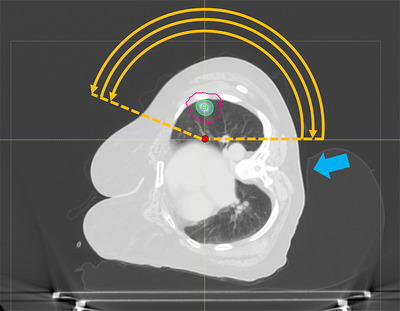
Planning CT dataset. The red dot indicates the shifted isocenter location. The shaded blue structure indicates the PTV, the 100% isodose line is shown in green and the 50% isodose line is shown in magenta. The orange arcs indicate the beam trajectories. The blue filled arrow indicates the immobilization device built‐up posteriorly.
